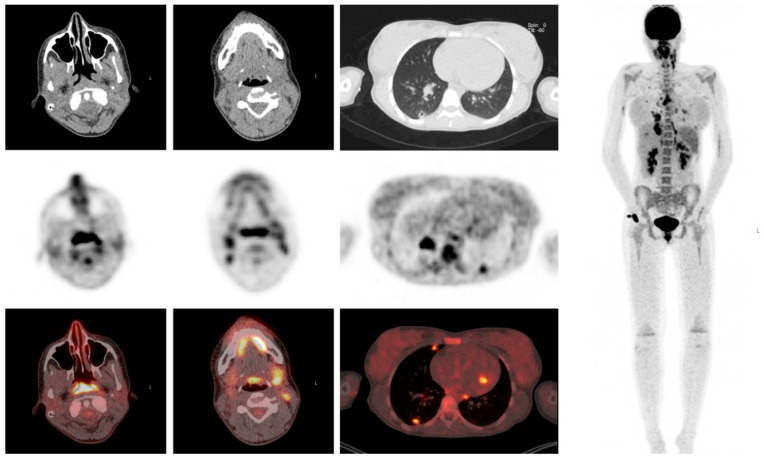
Clinical example 2. Intense FDG-uptake is visualized in the nasopharynx, tonsils, cervical and mediastinal lymph nodes and multiple pulmonary cavitating lung lesions.
